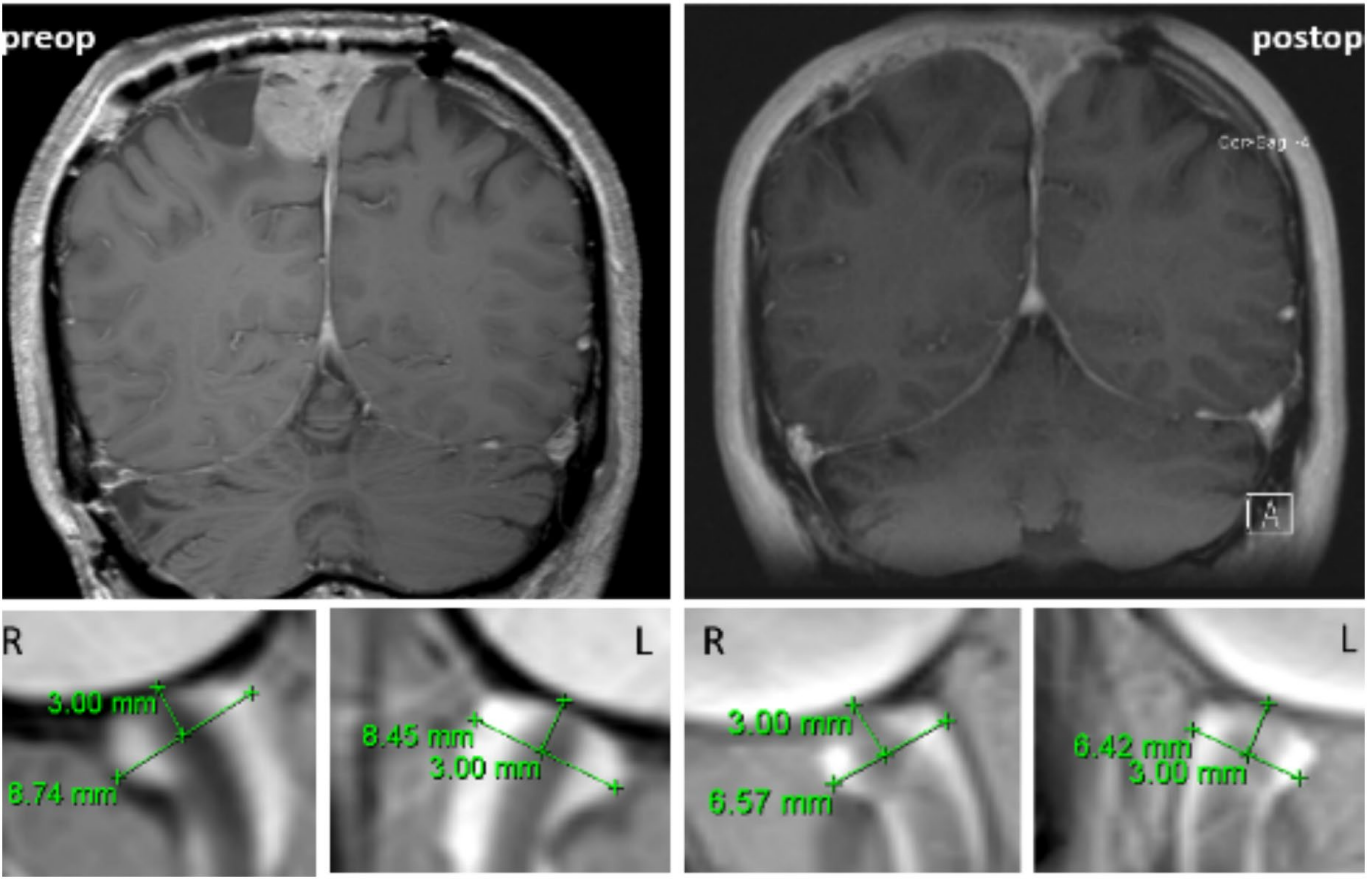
Example of an MRI-based pre- and postoperative ONSD measurement (lower row) in a study patient with a right-sided falcine meningioma and infiltration of the superior sagittal sinus with the corresponding MR imaging (upper row).
